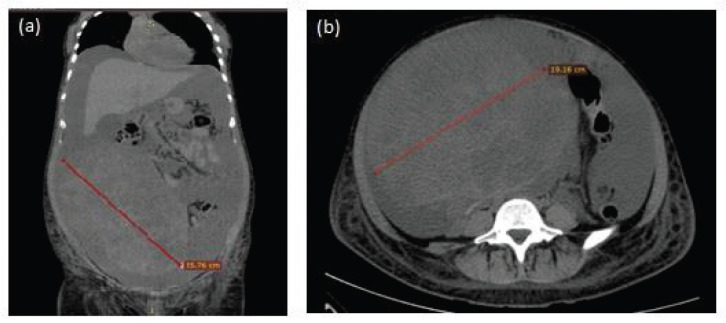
Representative images of simple abdominal computerised tomography (CT) scan. (a) Coronal section showing 25.76 cm (red line) heterogeneous abdominopelvic tumour and abundant ascites fluid. (b) Axial section with 19.16 cm tumour (red line).
